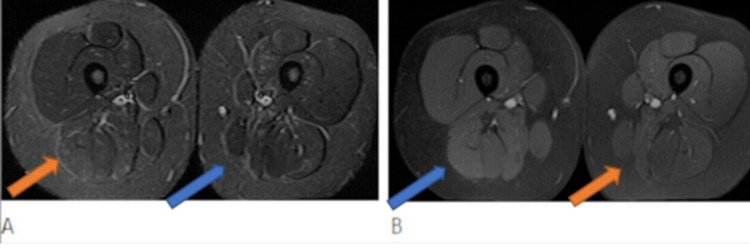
MR images of the lower thighs. Axial MR images of the lower thighs. A: STIR image demonstrates hyperintense signal in the muscles of posterior compartment. B: Post contrast T1 weighted image demonstrates subtle enhancement within the areas of signal abnormality of the muscles.
